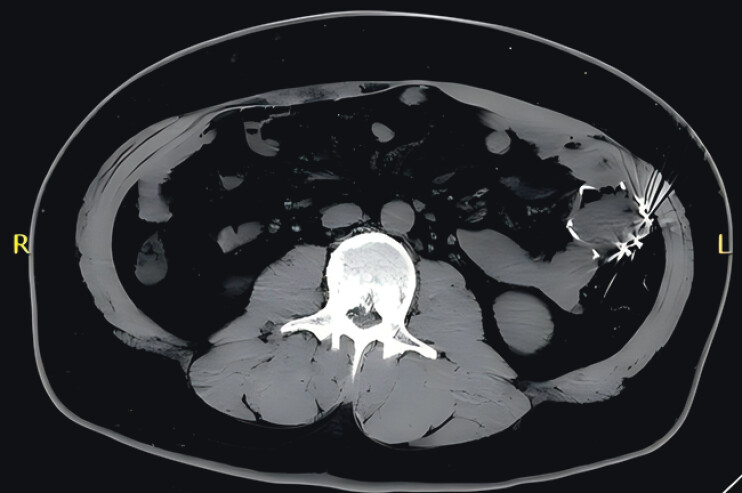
Computed tomography scan showed the stent had migrated to the jejunum.
